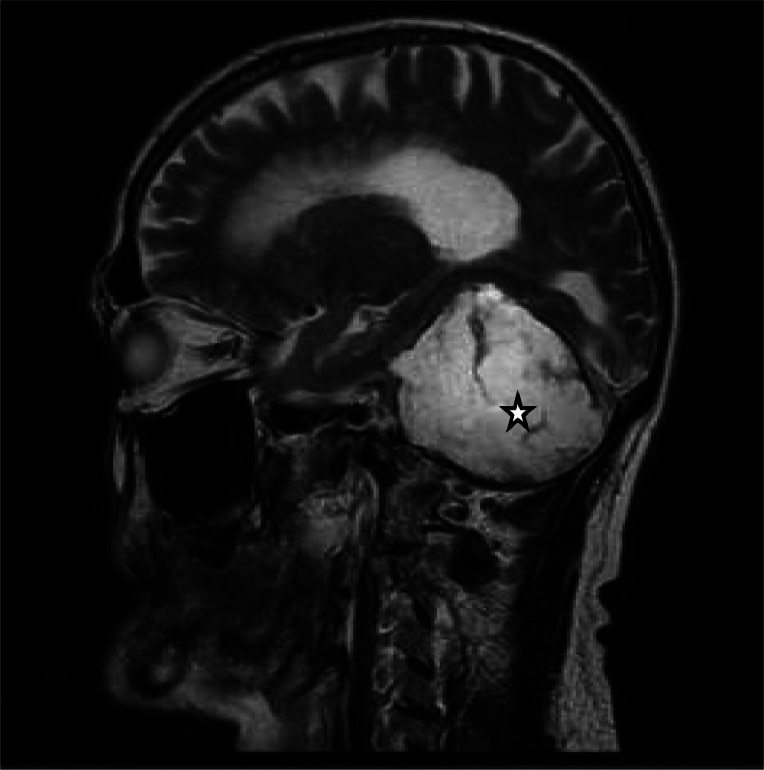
The sagittal view of T2 head MRI shows the hyperintense lesion (star sign).
